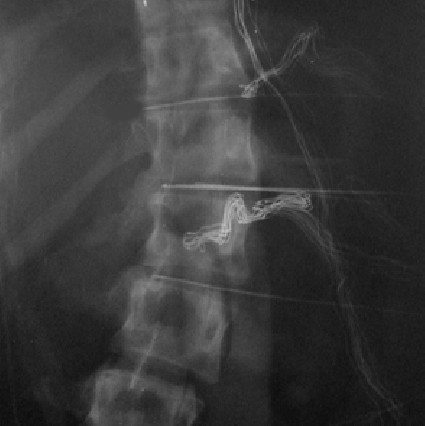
Part of intraoperative representative radiograph showing electrodes and pressure transducer inserted from the convex side of the scoliotic curve (same patient as figure 1).
